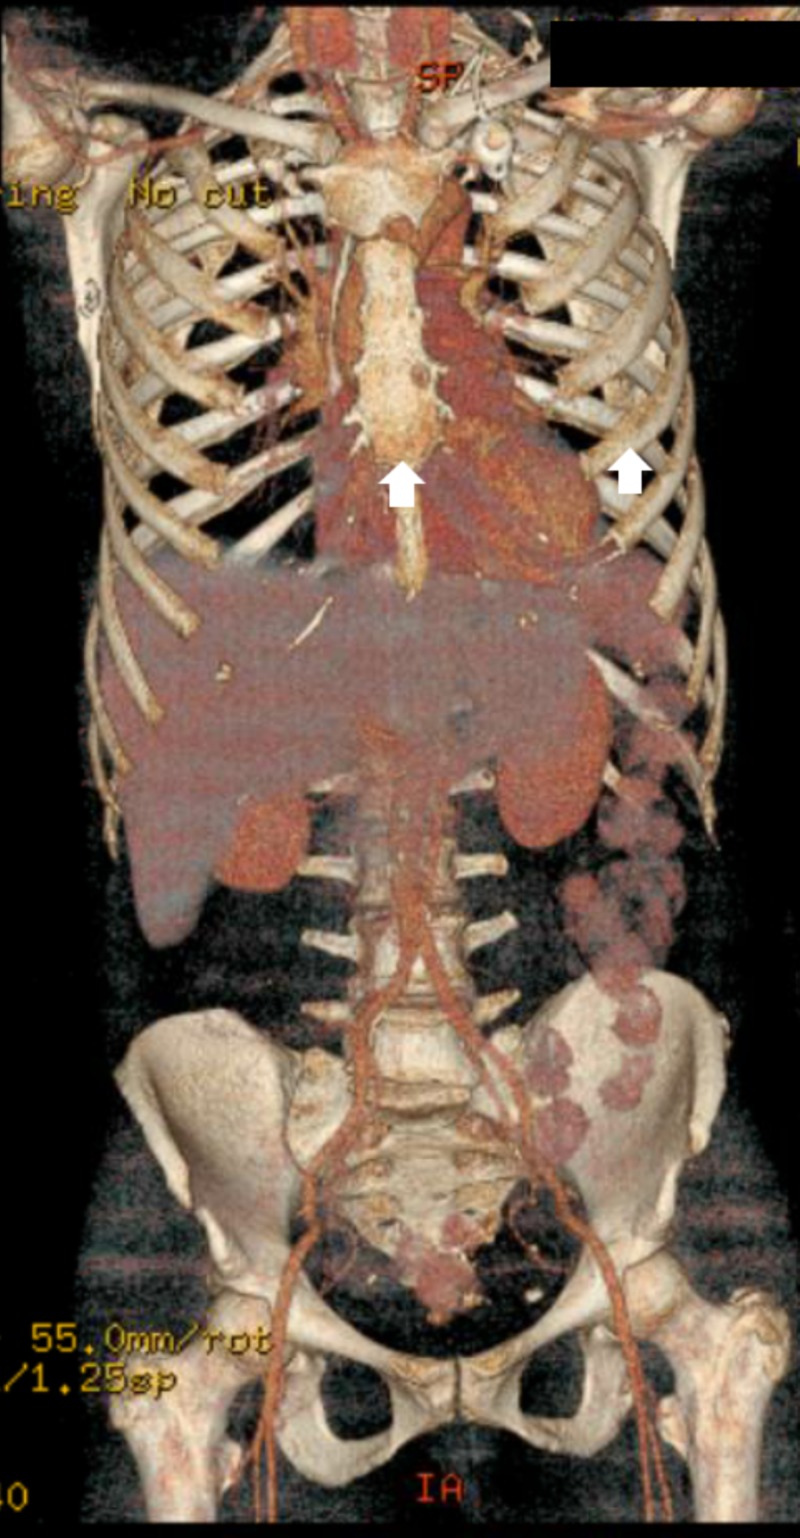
PET-CT scan for staging A PET-CT scan for staging showed diffuse bone involvement in the sternum, ribs (depicted with white arrows) and pelvic bones, no visceral disease.
